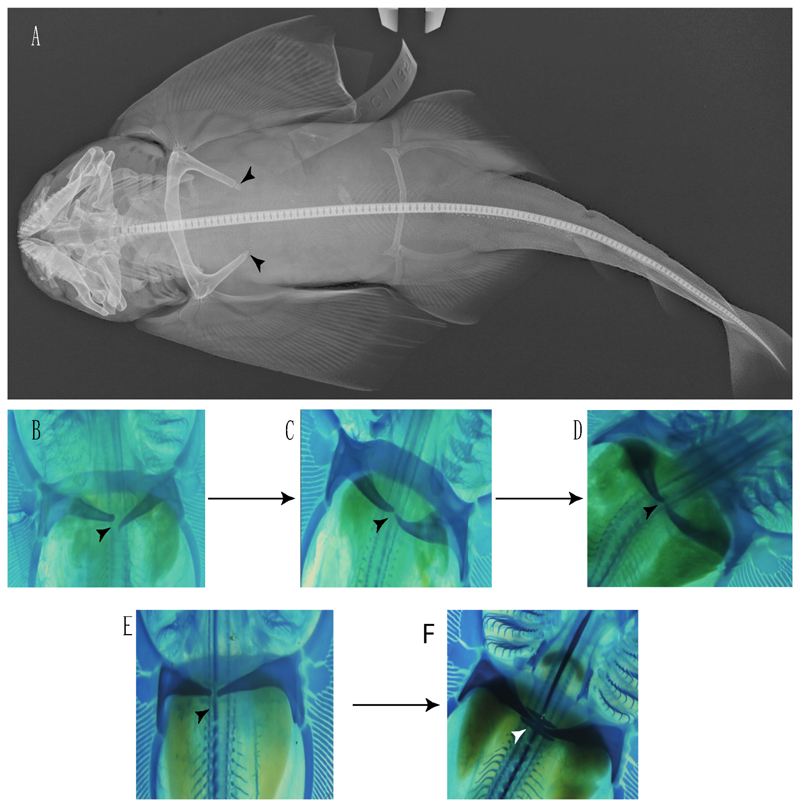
Dorsal view of the scapular region. State (0): (A) X-ray of a juvenile of *Squatina dumeril* [104] photo by Sandra J. Raredon. State (1): (B-F) Developmental stages of *Zapteryx brevirostris* (UREJ Unpublished data). Arrowheads: suprascapula cartilages.
